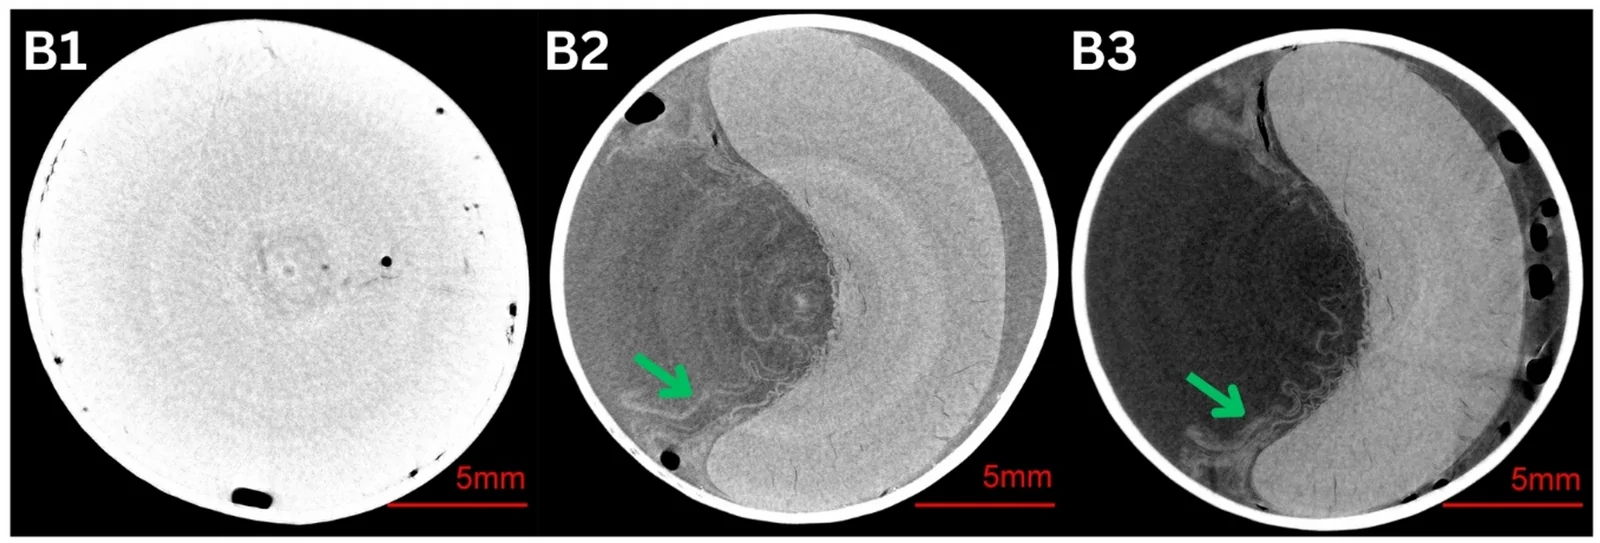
Axial CT slices of a sample container containing B1: Formalin fixed SDFT submerged in formalin, B2: Formalin fixed, 70% ethanol dehydrated SDFT submerged in 70% ethanol and B3: formalin fixed, dehydrated to 100% ethanol SDFT, submerged in 100% ethanol. Scale bars = 5 mm. Green arrow denotes loose connective tissue.
